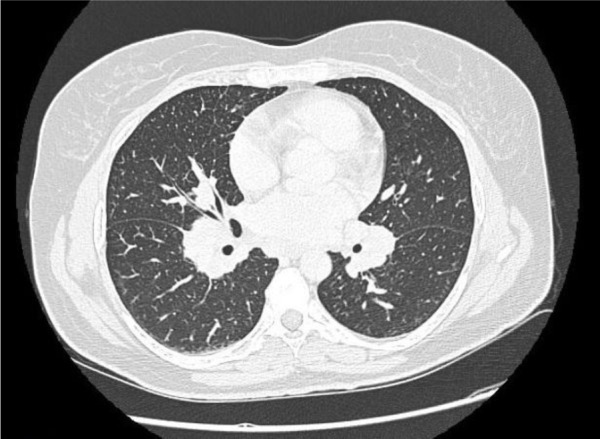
Spinal CT scan of Thorax with IV contrast showing systemic hilar and mediastinal adenopathies with subtle reticulonodular lungs infiltration compatible with sarcoidosis.
